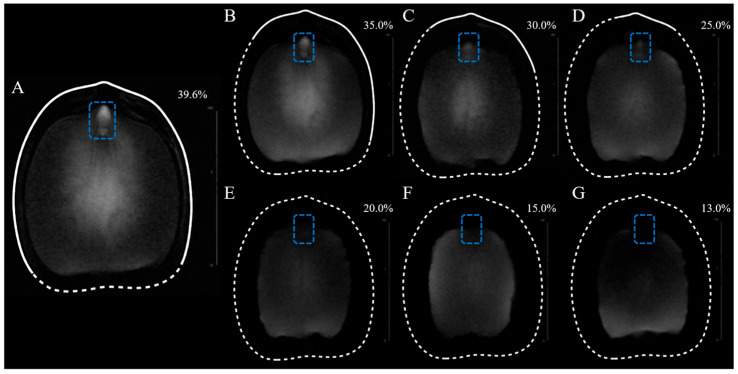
MRI images of *Quercus texana* seeds at different water content. (A–G) represents the seed imaging with water content of 39.6%, 35.0%, 30.0%, 25.0%, 20.0%, 15.0%, 13.0%, respectively. A white line was used to outline the seed’s initial shape. Solid lines represent seed coats containing water, while dashed lines denote seed coats devoid of water. The radicle region is outlined with a blue box.
